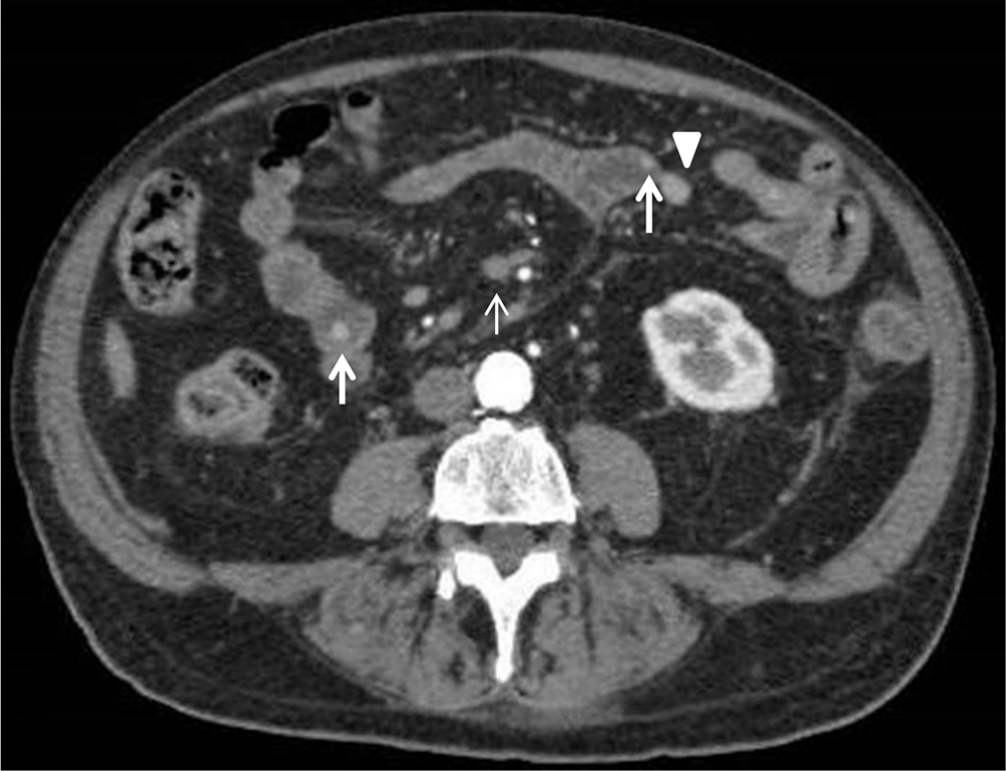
Abdominal CECT. Nodules in small bowel (arrows) with early intense enhancement followed by progressive washout. Peritoneal nodules (arrowhead) and mesenteric lymph nodes (thin arrow). CECT, contrast enhanced CT.
